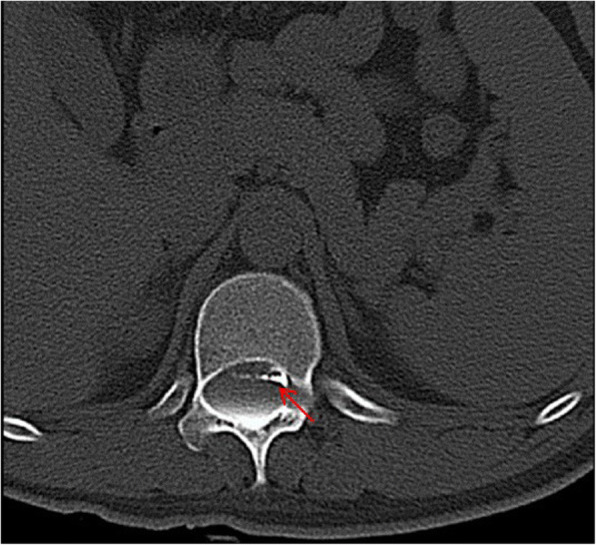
High resolution computed tomography: a funnel-shaped enhancement (red arrow) between the lower edges of the T12 body and the left nerve root
